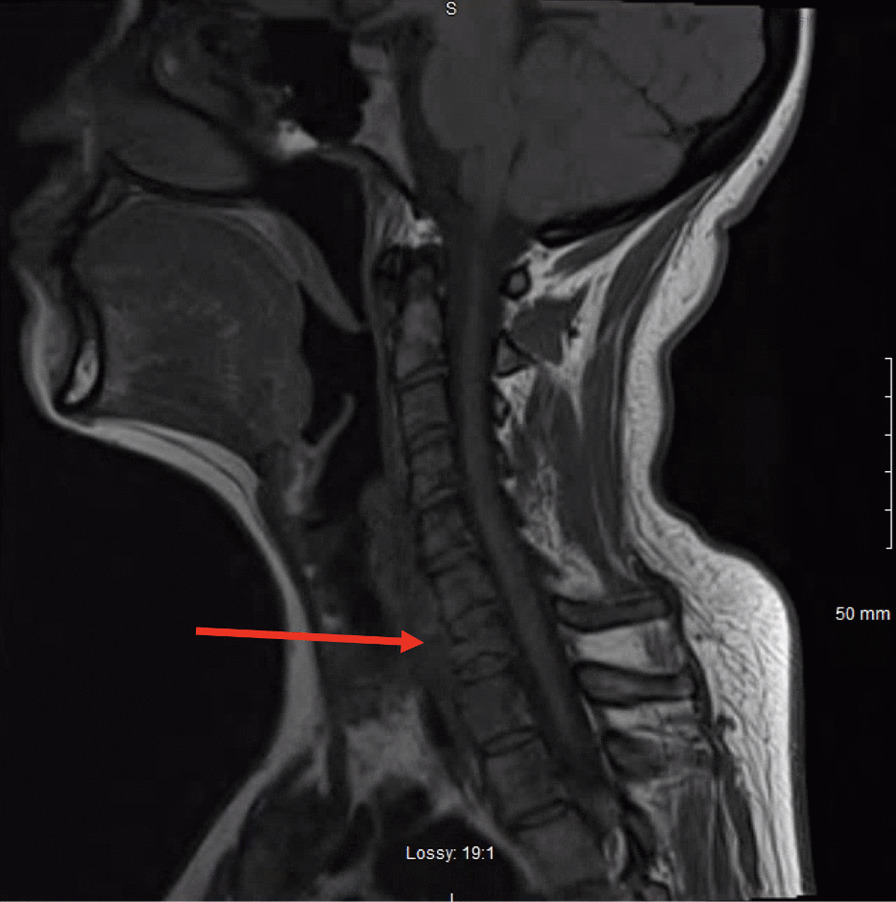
Sagittal section of a spine magnetic resonance imaging demonstrating a compression fracture at the level of C7 (red arrow)
